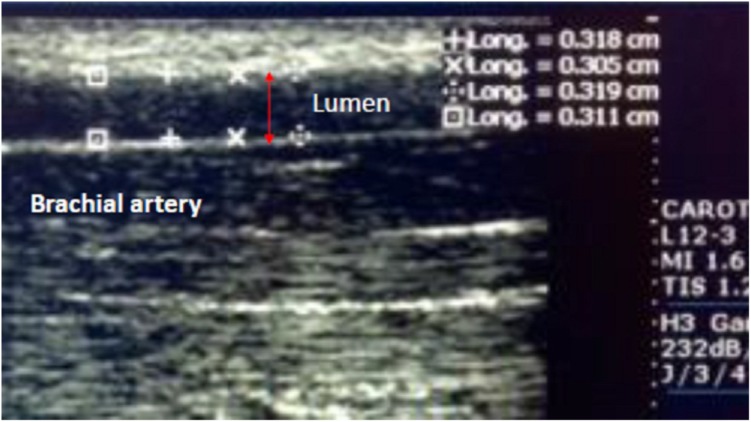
Shows a segment of the artery located at the anterior and posterior portions of the intima. This image was obtained by using grayscale bidimensional ultrasound over a longitudinal plane to the artery.
